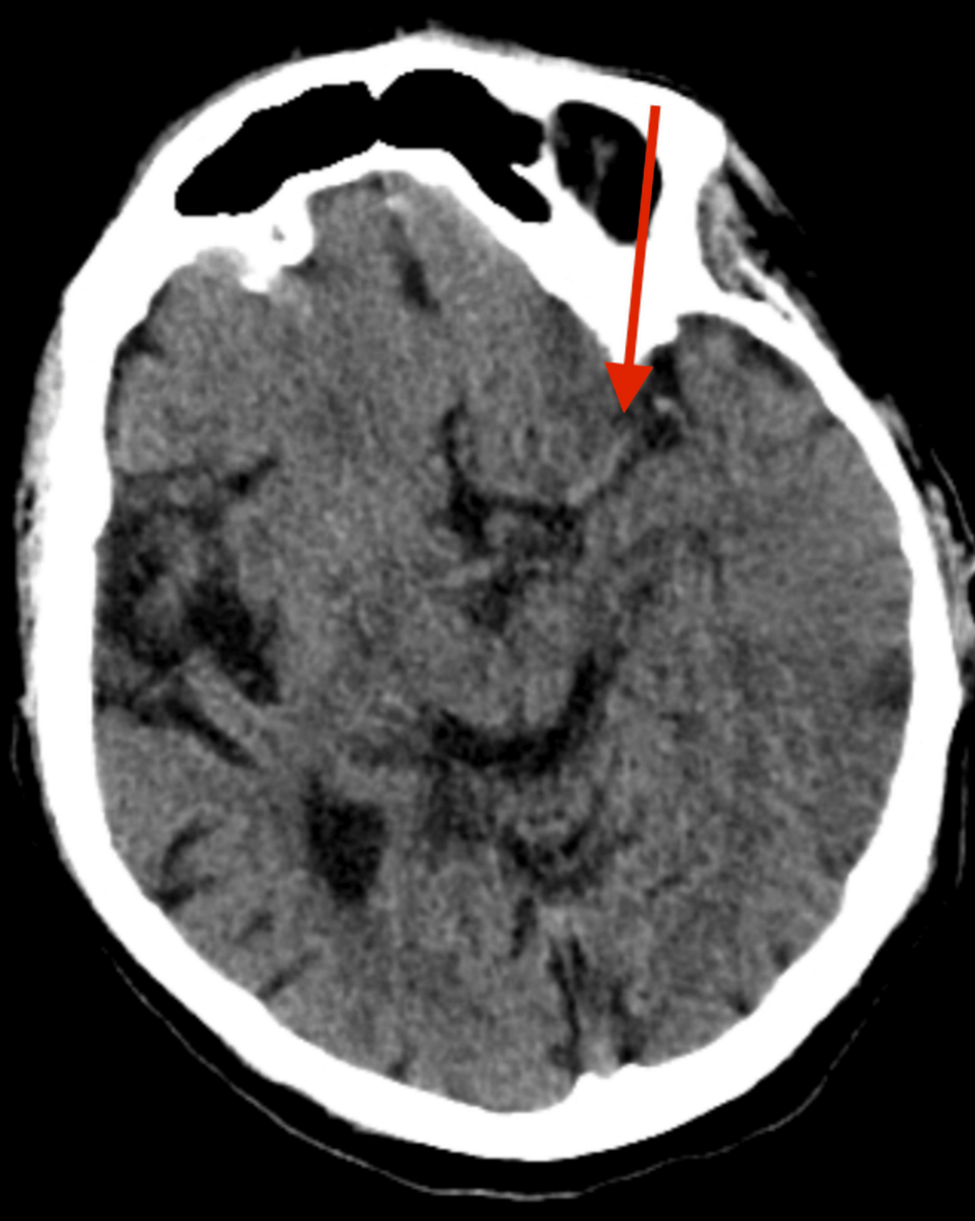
Computed Tomography (CT) Head Showing Left M1 Middle Cerebral Artery (MCA) Hyperdensity Plain CT head showing a hyperdense thrombus (red arrow) within the left M1 segment of the middle cerebral artery. Note the radiological evidence of a prior left frontal infarct.
